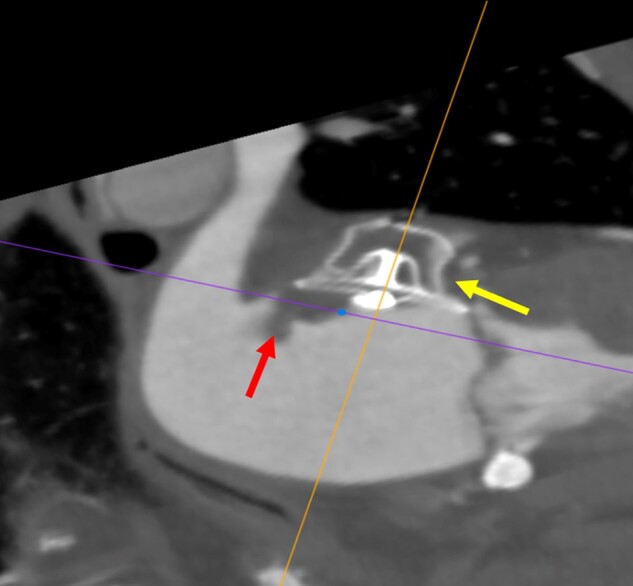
Device-related thrombosis (DRT) after LAA occlusion in a patient implanted with an AMULET device. The 3-month follow-up CT scan shows the AMULET device in a good position (leftward pointing arrow) with a large thrombus on the device disk (upward pointing arrow).
